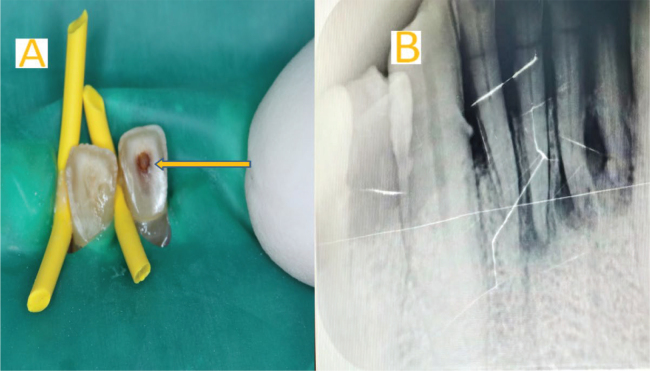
Images prior to root canal treatment. (A) Clinical photograph during endodontic treatment of teeth #31 and #41, demonstrating standard operative field setup; (B) Preoperative periapical radiograph showing pulp chamber morphology prior to instrumentation.
